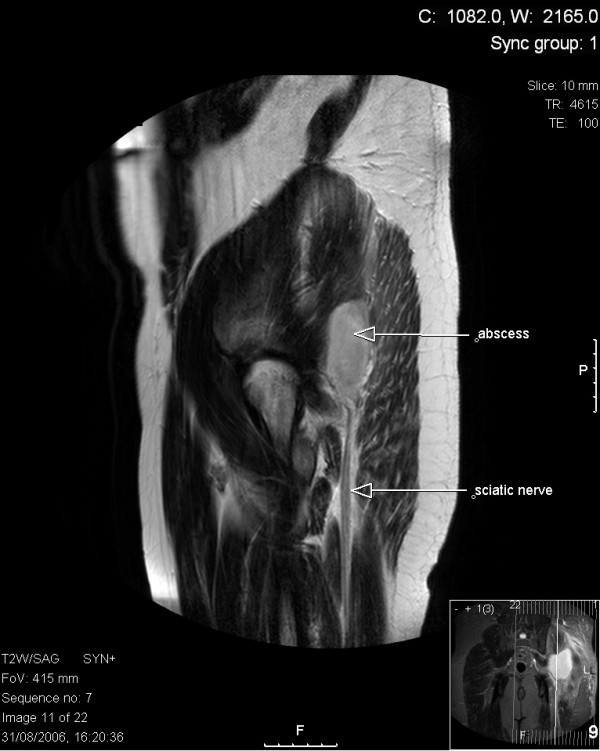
Axial T2 magnetic resonance imaging section through the hip region showing abscess collection in relation to the left sciatic nerve.
